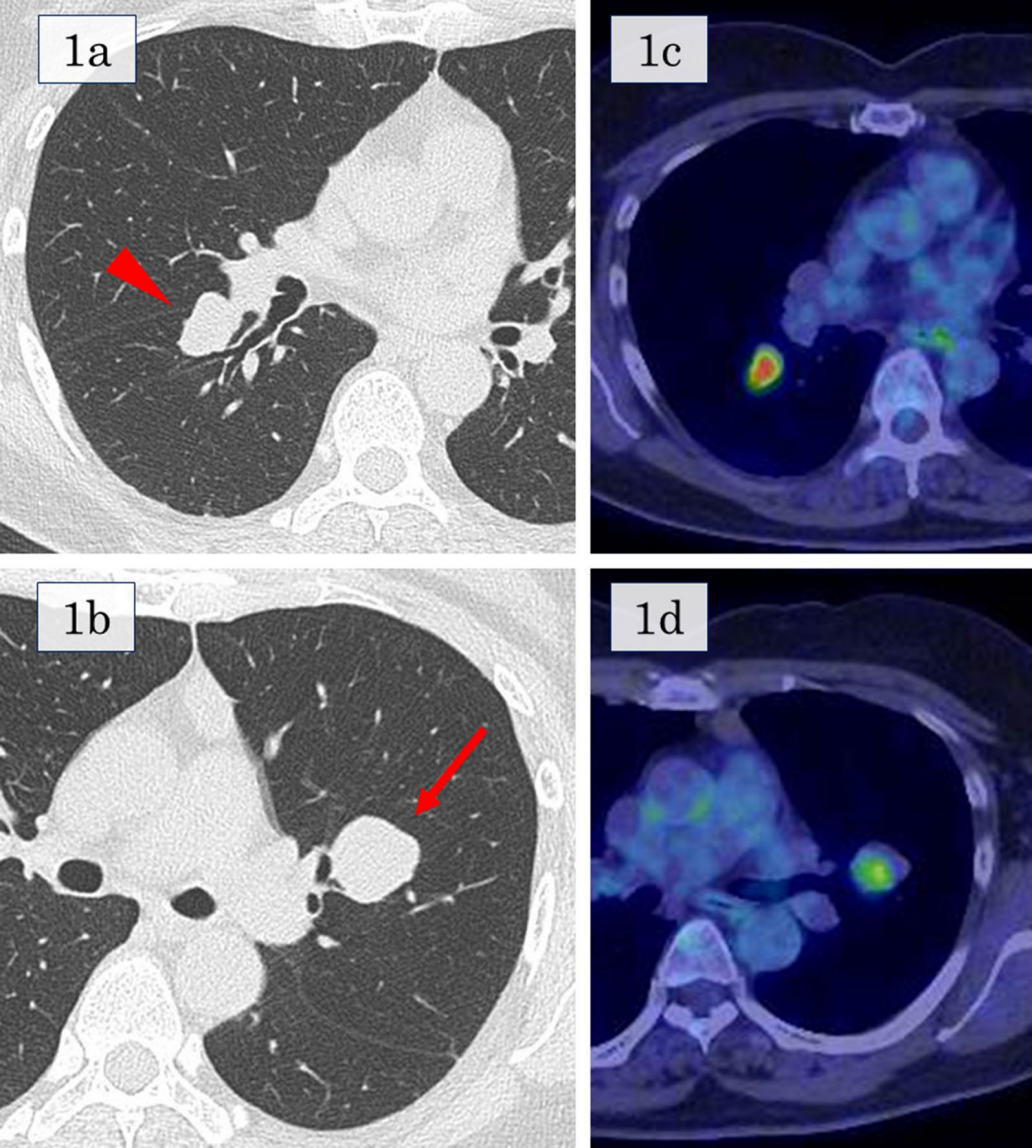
Computed tomography and 18F-fluorodeoxyglucose positron emission tomography findings. a A solid nodule (maximum diameter, 2 cm) located centrally in the right lower lobe. b A solid nodule (maximum diameter, 3 cm) located centrally in the left upper lobe. c Maximum standardized uptake value of 8.3 corresponding to the nodule in the right lower lobe. d Maximum standardized uptake value of 5.7 corresponding to the nodule in the left upper lobe
